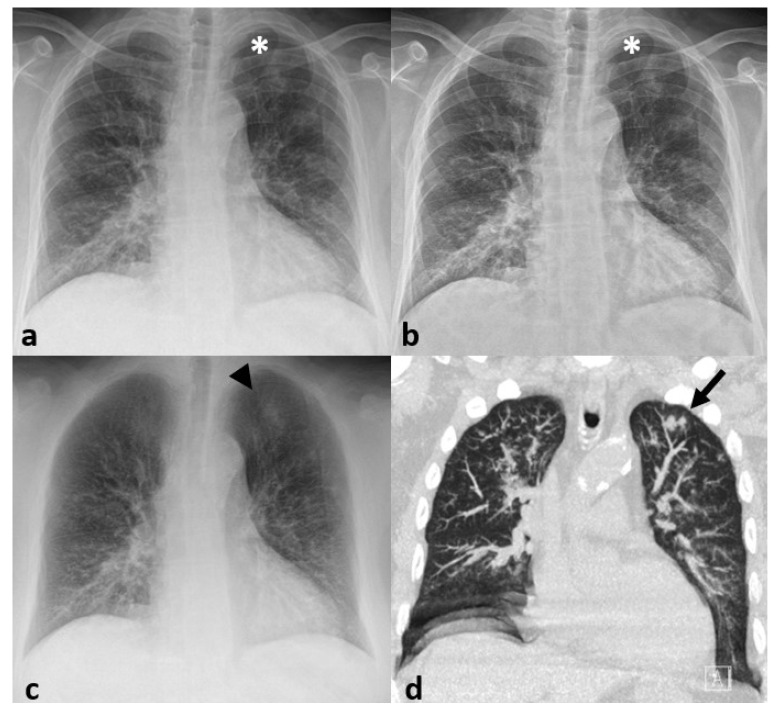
62-year-old male COVID-19 patient presenting with patchy consolidation in the left upper lobe on (d) chest computed tomography (arrow). These differences are not readily seen (a) in the conventional chest radiograph and (b) in the enhanced images (asterisks) and could also be due to a summation artifact of the ribs. Opacities become visible when bone was subtracted in the (c) bone suppressed images (arrowhead).
